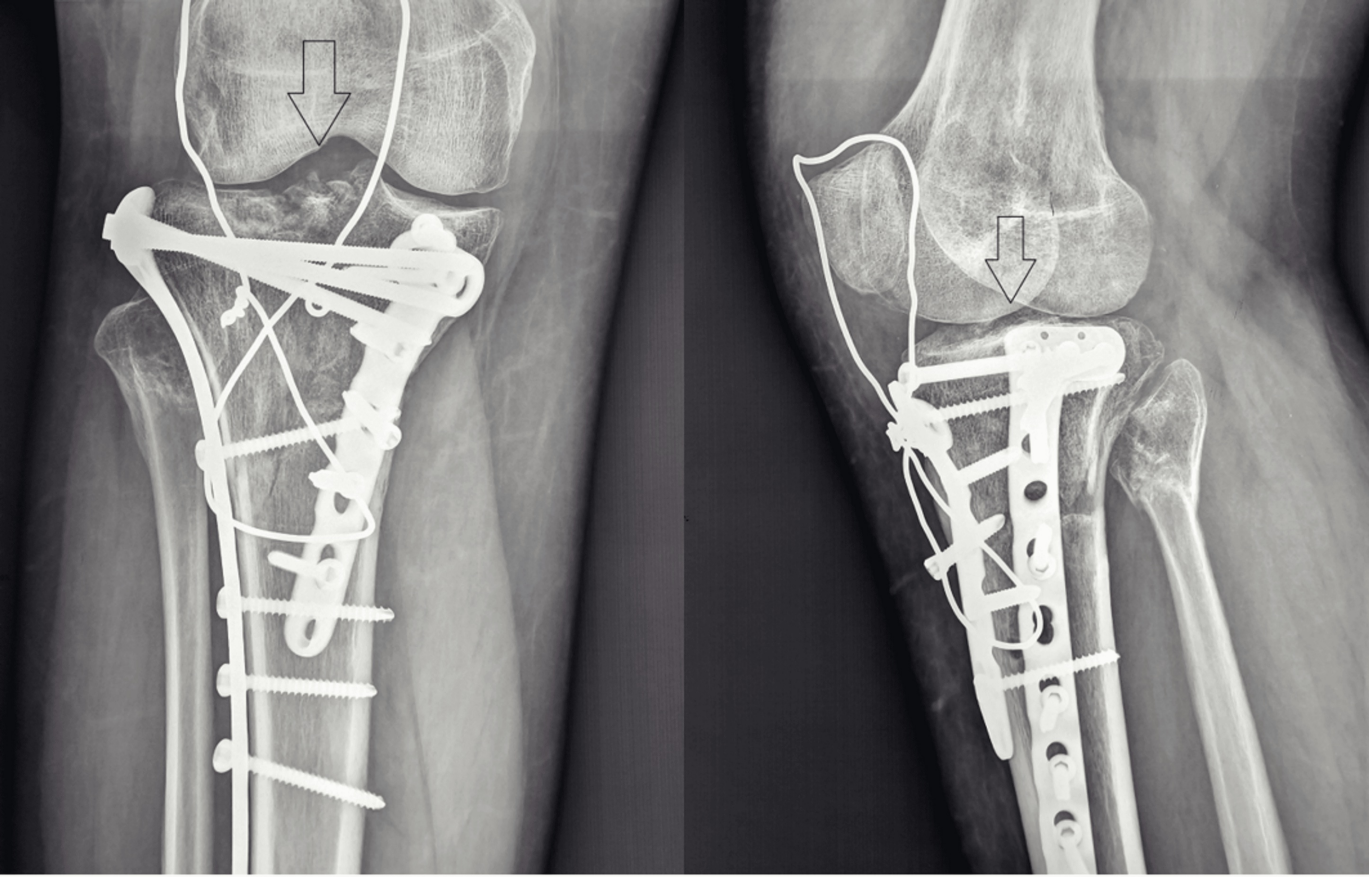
Postoperative X-ray at the one-year follow-up
